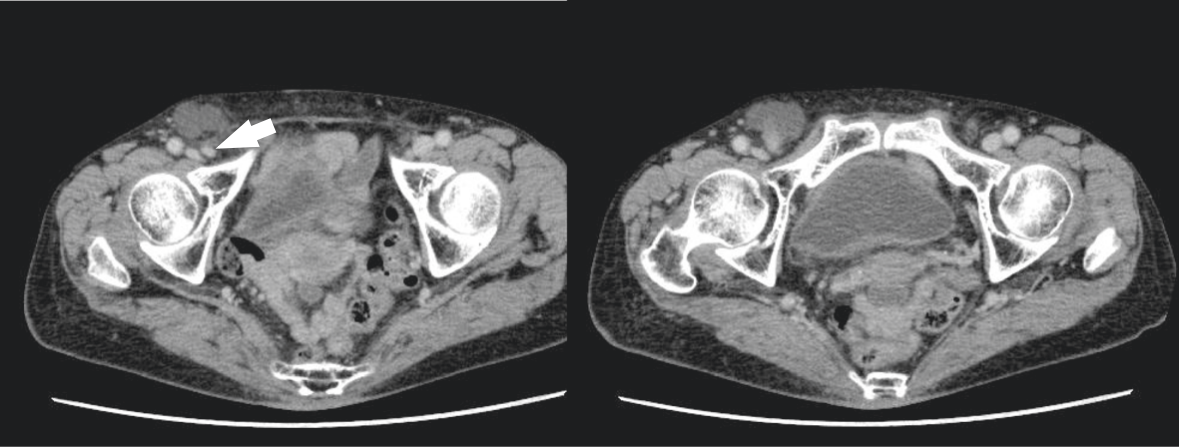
Preoperative findings of abdominal contrast-enhanced computed tomography. Abdominal contrast-enhanced computed tomography demonstrates a 4 × 3 cm cystic structure and enhanced cord-like structure (*arrowhead*) in the right groin region without the small bowel
